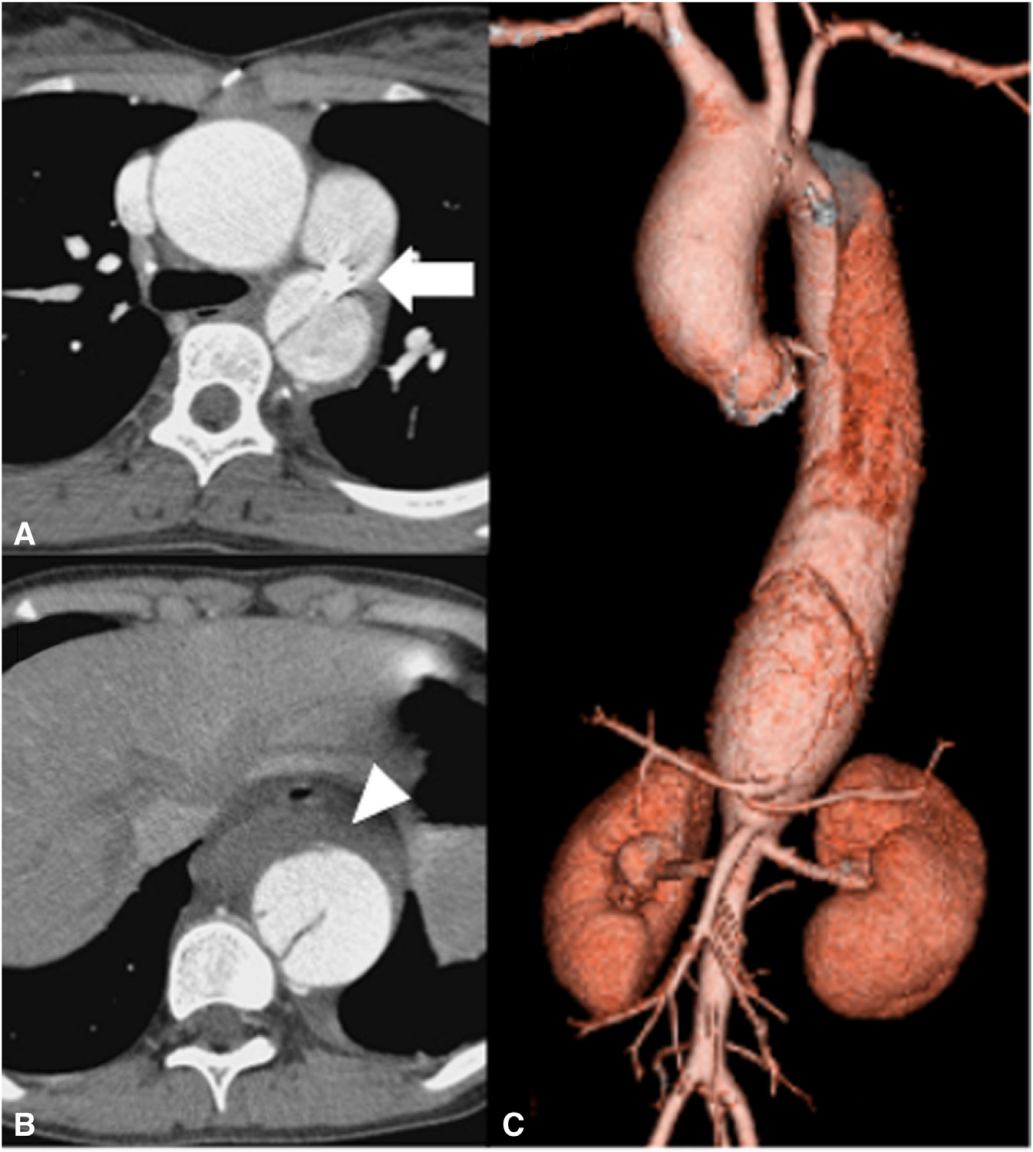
Computed tomography angiography on admission. A, Ascending aortic aneurysm (43 mm) and patent ductus arteriosus with the coil used for previous intervention (*arrow*). B, Type B aortic dissection with the entry at middle descending aorta surrounded by a low-density area (*arrowhead*). C, Three-dimensional computed tomography showing extensive aortic disease with ascending aortic aneurysm and type B aortic dissection.
